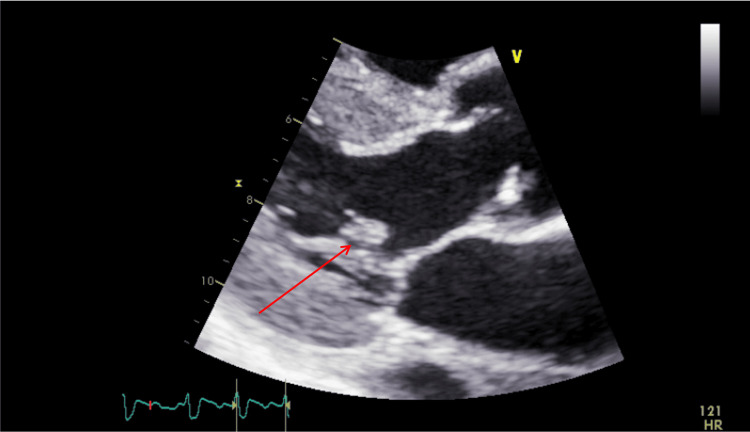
Mitral valve vegetation (red arrow) seen on echocardiogram on day three.
